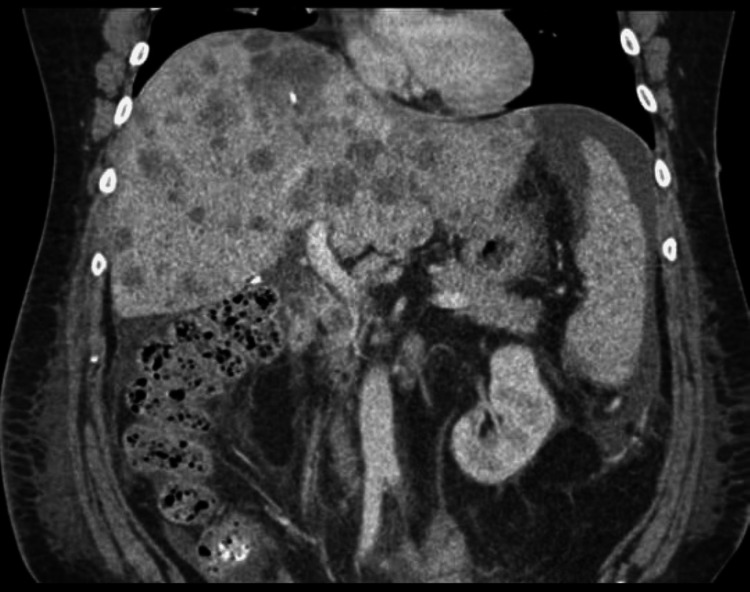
Radiograph showing liver metastasis
